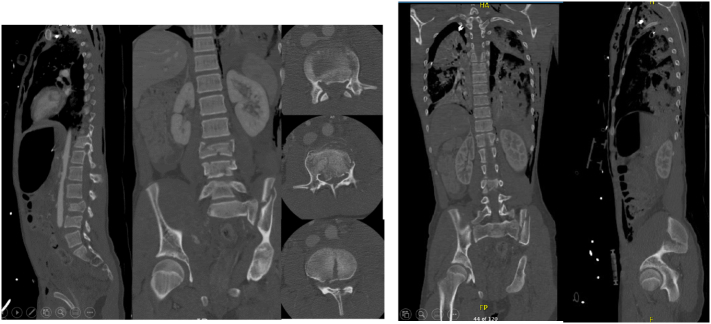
CT scan of the lumbosacral spine shows complete burst fracture of the L3 vertebral body extending into both pedicles, with significant 1.2 cm osseous retropulsion within the canal and resultant compression of the cauda equina nerve roots, further burst fracture of the L 4 vertebral body and minimally displaced transverse process fractures of the right L1-L4 vertebrae.
